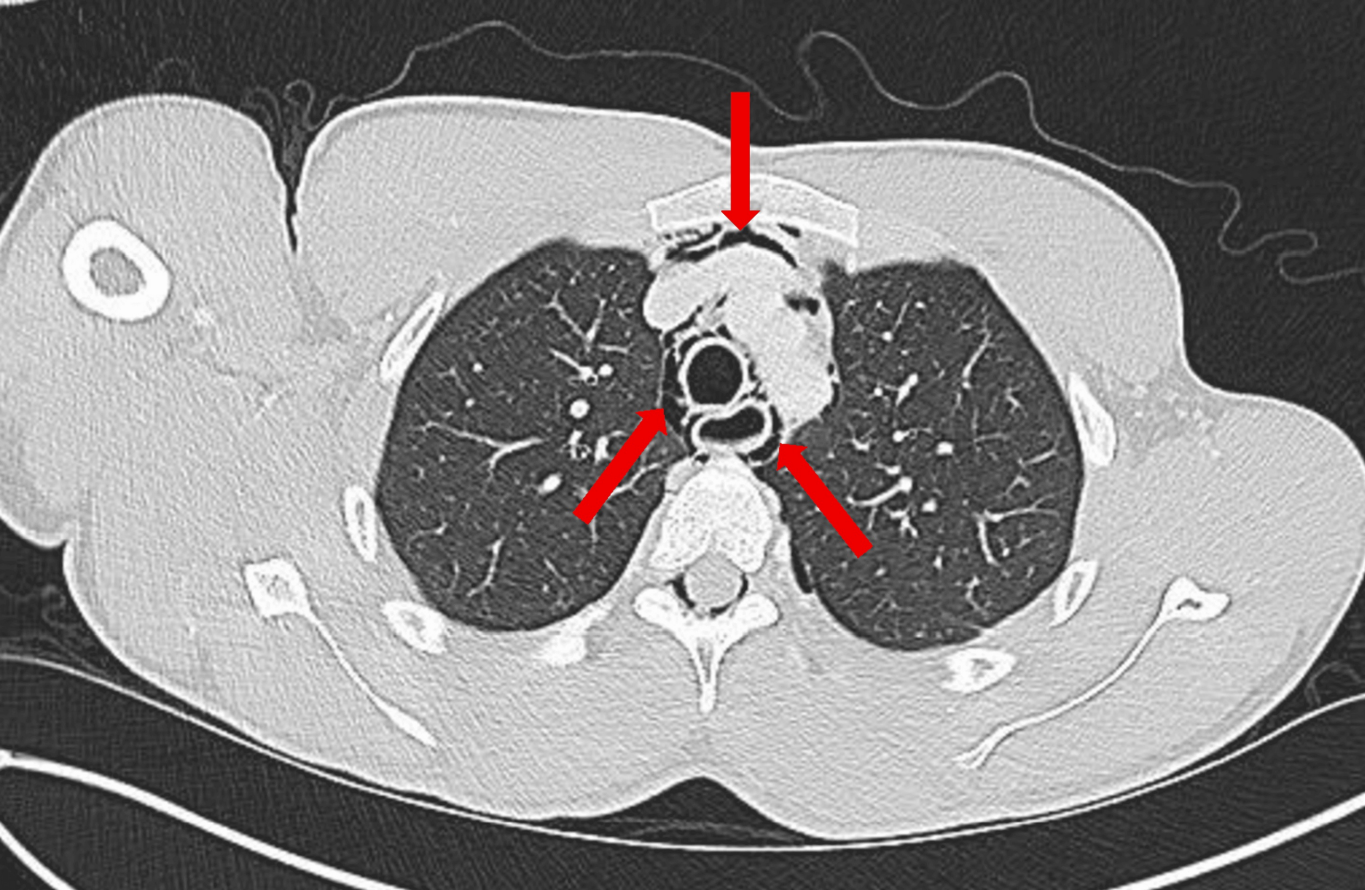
Computed Tomography (CT) scan of the chest (axial view) demonstrating moderate pneumomediastinum (red arrows).
